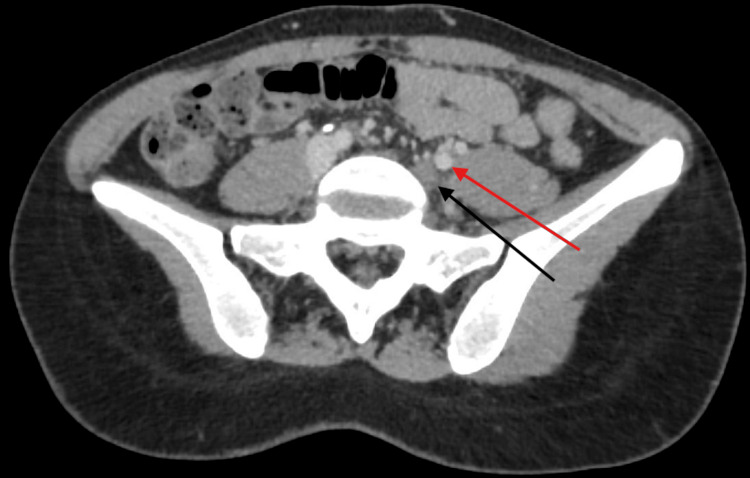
Computed tomography (CT) venogram showing acute thrombosis involving the left iliac vein (black arrow) with compression of the left common iliac vein by the overlying right common iliac artery (red arrow) against the lumbar spine and findings consistent with May-Thurner syndrome.
